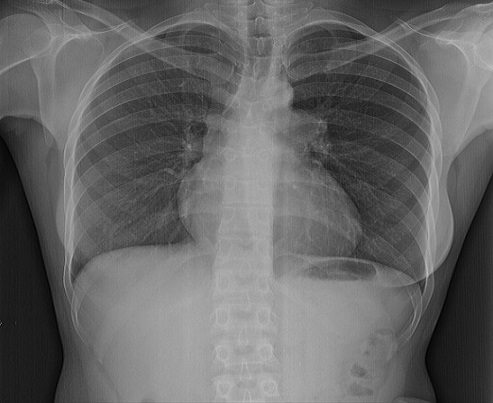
Radiographie télécœur de face montrant une cardiomégalie modérée (index cardio- thoracique à 0, 53), une convexité de l'arc moyen gauche, une vascularisation pauvre en périphérie. Dilatation de la partie proximale de l'artère pulmonaire gauche
